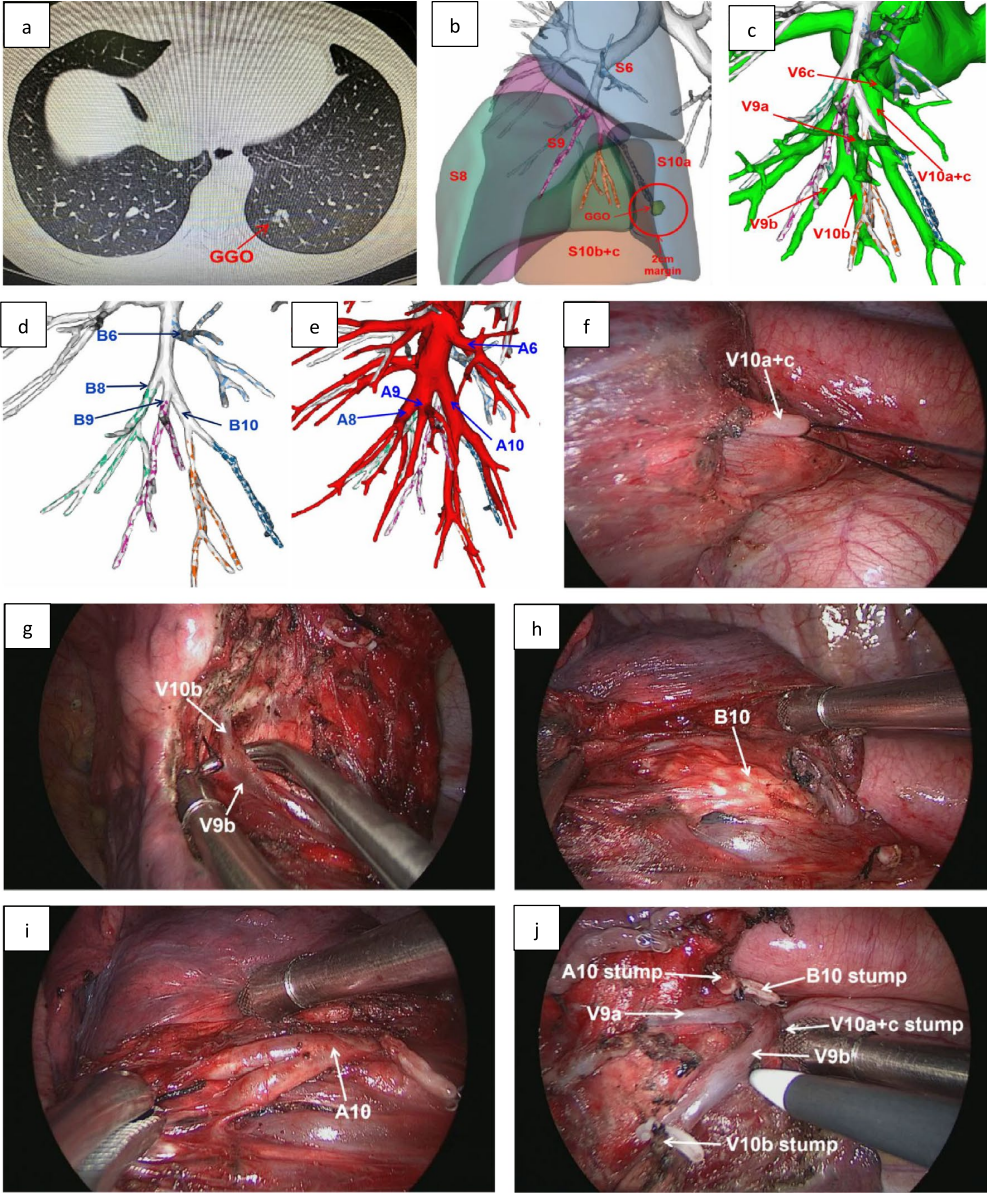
The CT scan and 3D-CTAB of a 48-year-old female patient. One GGO was found in the left lower lobe. According to the 3D-CTAB, anatomical segmentectomy with left S10 was performed; a the CT scan showed that a GGO was located in the left lobe (S10); b according to the results of 3D-CTAB and the safe margin of 2 cm, the confirmed target lung segment was LS10; c 3D-CTAB showed pulmonary vein anatomy; d 3D-CTAB showed the pulmonary bronchial anatomy; e 3D-CTAB showed the pulmonary artery anatomy; f V10a + c was showed in the operation; g V9b and V10b was showed in the operation; h B10 was showed in the operation; i A10 was showed in the operation; j the surgical wound after LS10 segmentectomy
